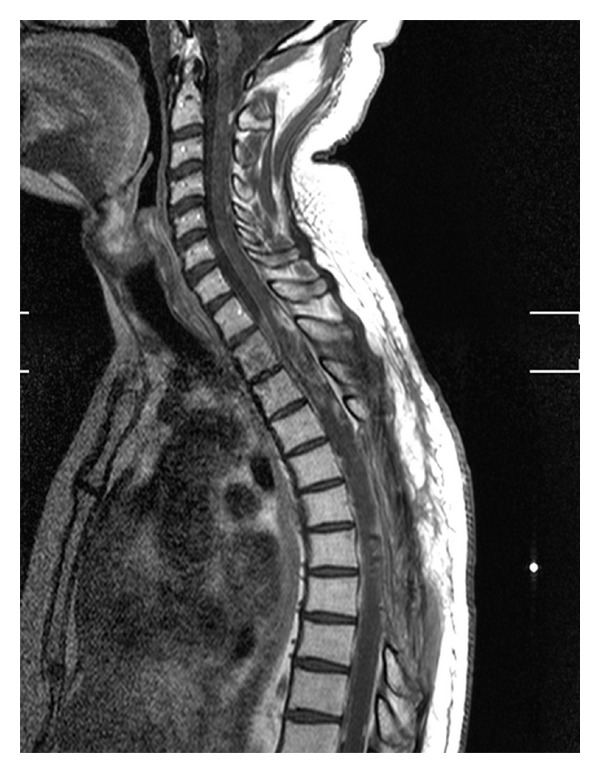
Posttreatment cervicodorsal MRI: we can notice a remarkable improvement of the spinal infiltration.
